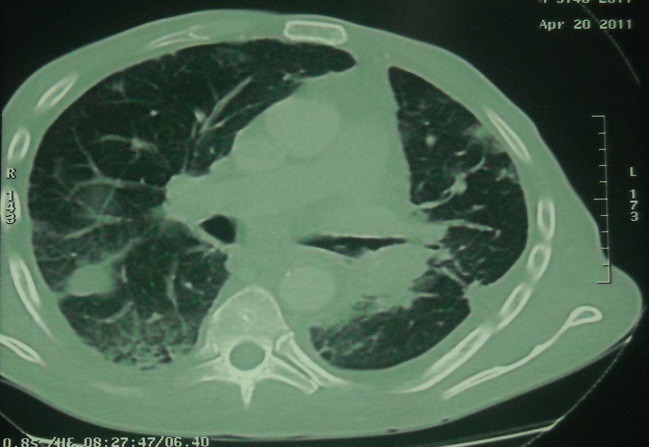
Thoracal CT showed mass lesion in right lower lobe
